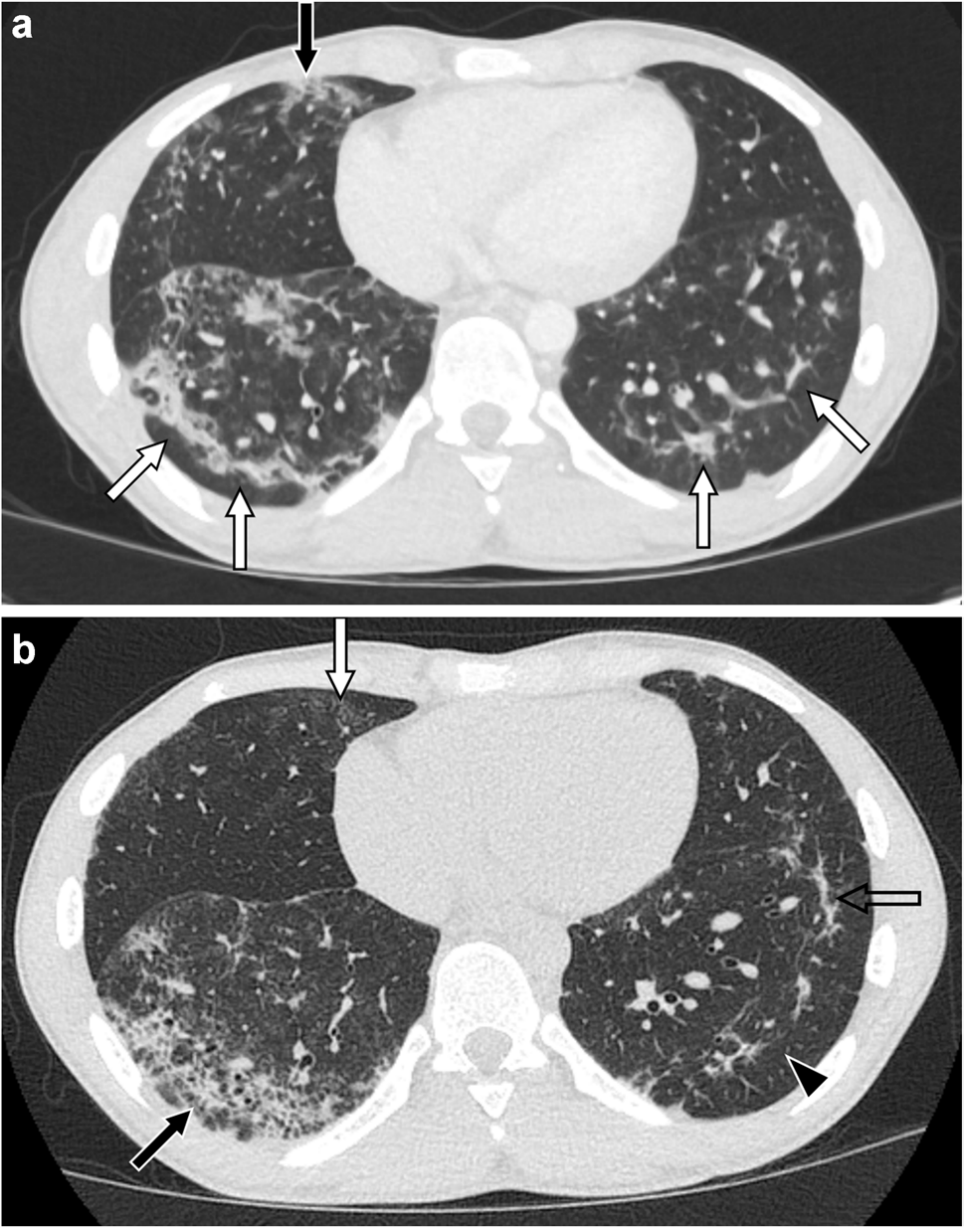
An 18–year-old male who developed dyspnea and dry cough over the course of several weeks while receiving chemotherapy that included bleomycin for testicular mixed germ cell tumor. He had a history of cigarette smoking and vaping nicotine, but quit during treatment, and did not exhibit clinical evidence of infection at the time of evaluation for his respiratory symptoms. a Axial CT image with contrast showing peripheral consolidation in the right middle lobe (*black arrow*) and bilateral peripheral perilobular opacities (*white arrows*) typical of organizing pneumonia. b Axial CT without contrast obtained 2 weeks later showing peripheral consolidation and reticulation in the right lower lobe associated with bronchial/bronchiolar dilation (*black arrow*), mild ground-glass opacity remaining at prior site of right middle lobe consolidation (*white arrow*), and areas of decreased (*arrowhead*) and increased (*open arrow*) perilobular opacity in the left lower lobe. A presumptive diagnosis of bleomycin-induced lung disease was made based on clinical presentation and imaging findings. His symptoms improved upon completion of chemotherapy and initiation of steroids, further supporting the presumptive diagnosis
